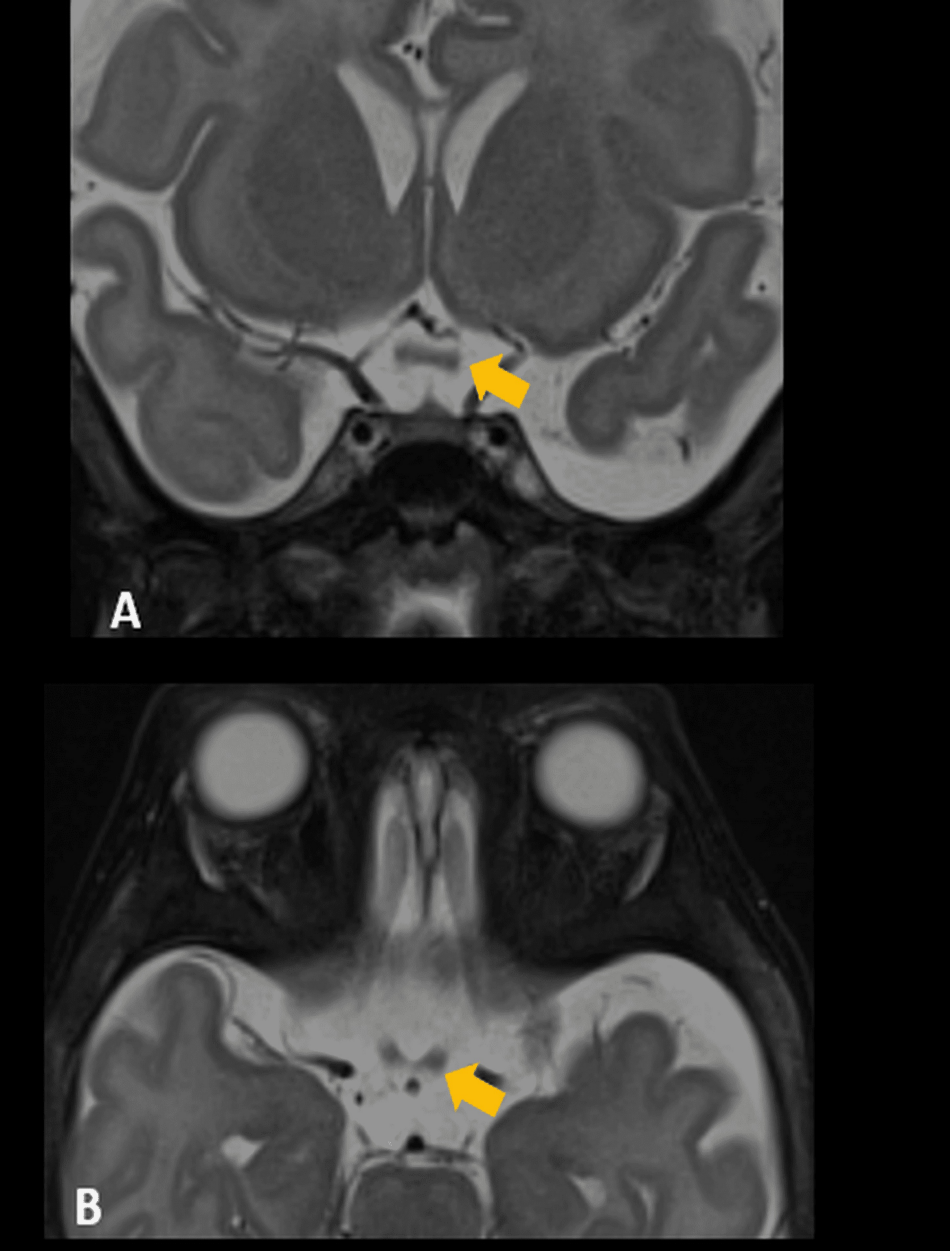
MRI T2 fat saturated sequence in coronal (A) and axial (B) sections shows thinning of the optic chiasm (arrows)
